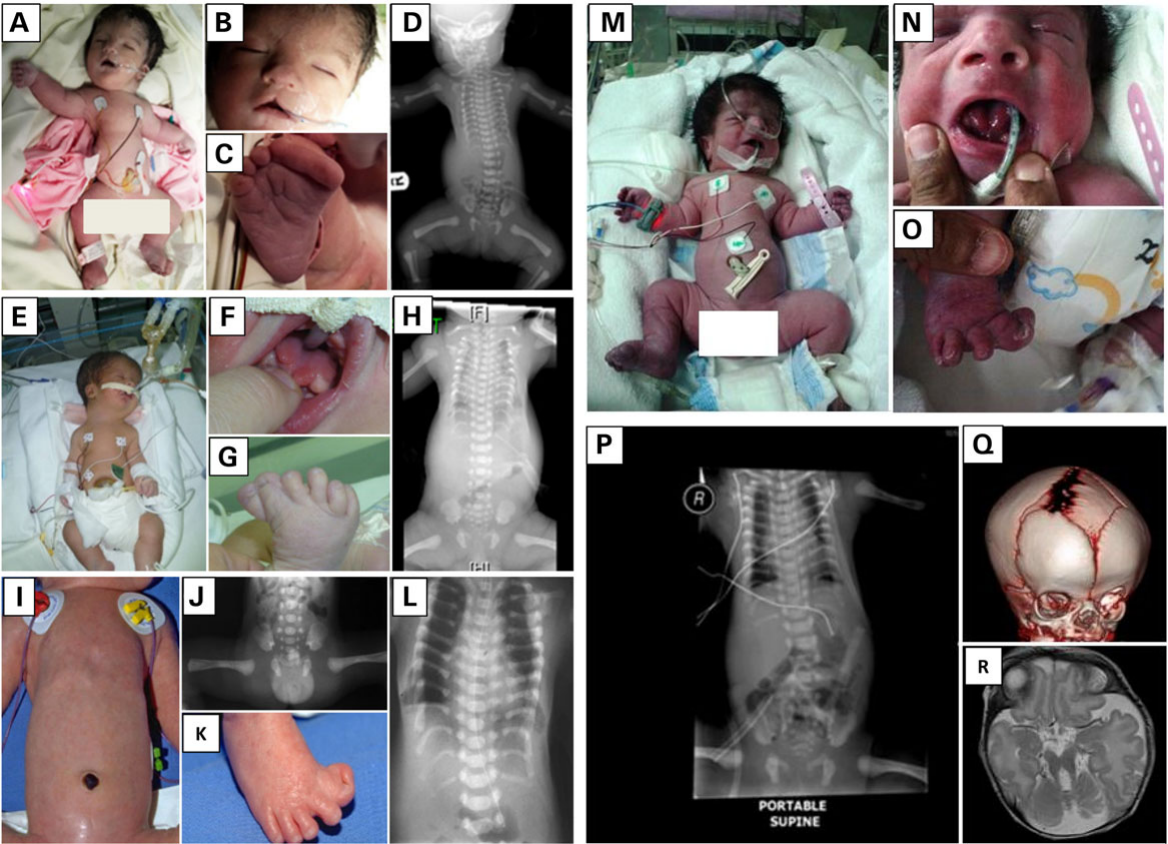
Clinical images of the study families. (A–D) Postnatal clinical photograph and radiological imaging of the index case from Family 1 showing severely narrow chest and thorax, mild cleft lip (notch), short limbs and synpolydactyly. (E–H) Postnatal clinical photograph and radiological imaging of the index case from Family 2 showing very narrow chest, short extremities, synpolydactyly, tongue hamartoma (lobulated tongue) and omphalocele. (I–L) Postnatal clinical photograph and radiological imaging of the individual Family 3_II:1 showing long and narrow thorax with short horizontal ribs, dysplastic pelvis with acetabular spurs and hexadactyly of the feet. (M–R) Postnatal clinical photograph, radiological imaging, 3D skull computed tomography and MRI of the index case from Family 4 showing coarse facies, midface hypoplasia, partially bifid tongue, polydactyly, short tubular bones, bell-shaped thorax with short ribs, unilateral coronal craniosynostosis with prominent and widened anterior and posterior fontanels, and brain vermian hypoplasia with molar-tooth appearance.
